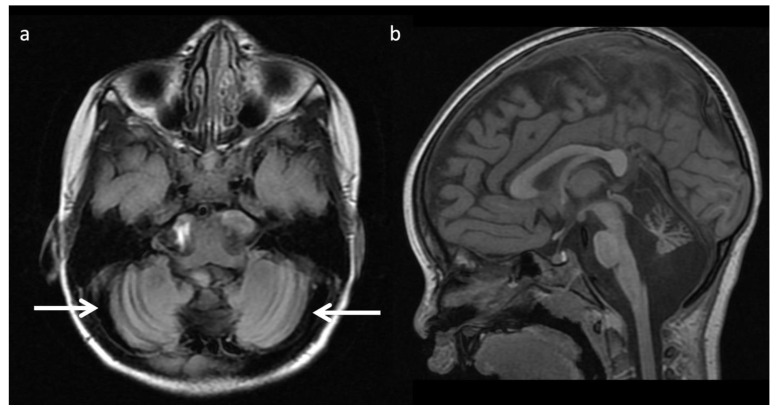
Three year old child with congenital disorder of glycosylation Type 1a (CDG-1a), which is an early-onset neurodegenerative disorder with selective hindbrain involvement. (a) Axial T2 FLAIR image of the cerebellum shows diffuse marked cerebellar atrophy and diffuse cerebellar hyperintense T2 signal (white arrow). (b) Sagittal T1 image again shows the marked cerebellar atrophy. The pons is also mildly hypoplastic.
